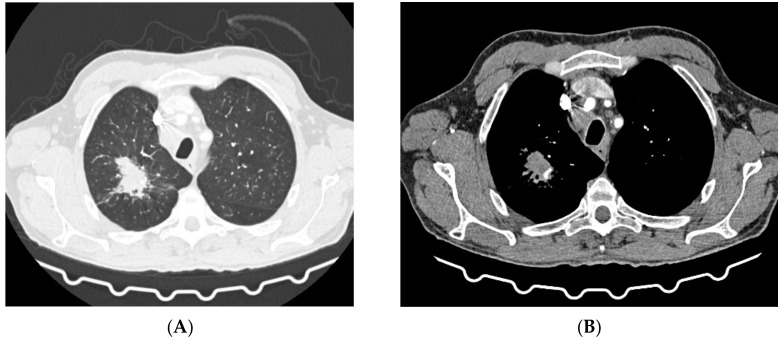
(A,B)—CT scans taken during the fifth month of treatment, September 2021.
